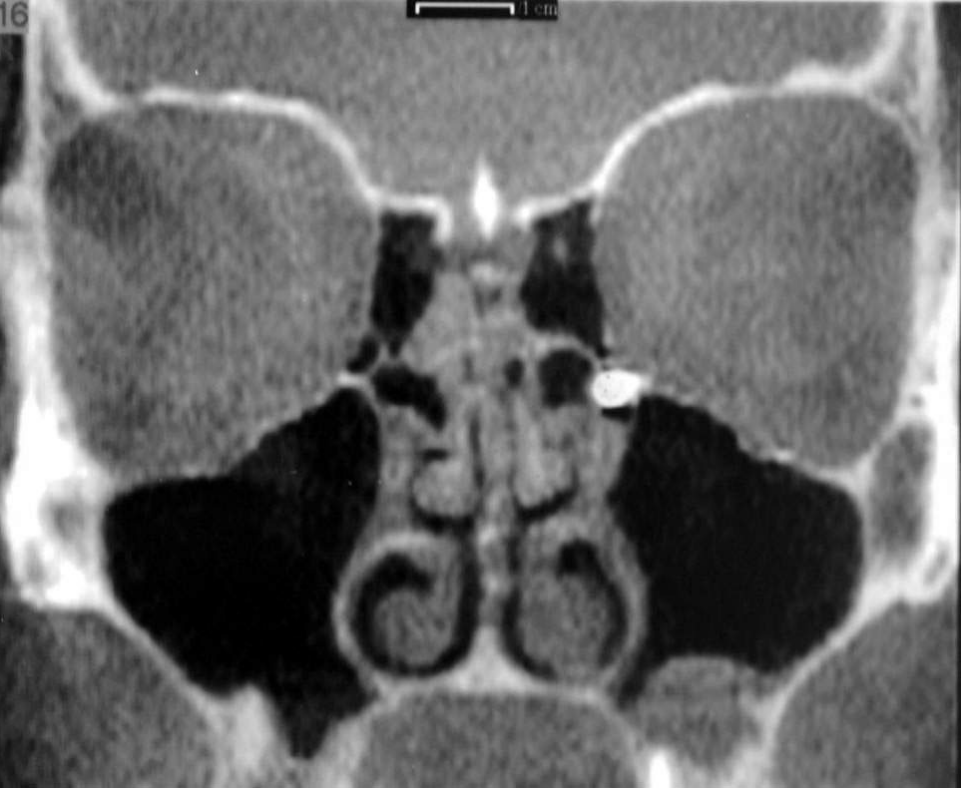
Computed tomography scan (coronal plane) showing the foreign body located in the supero medial aspect of the maxillary sinus and partial mucosal thickening of the sinus upon the roots of the upper first molar.
